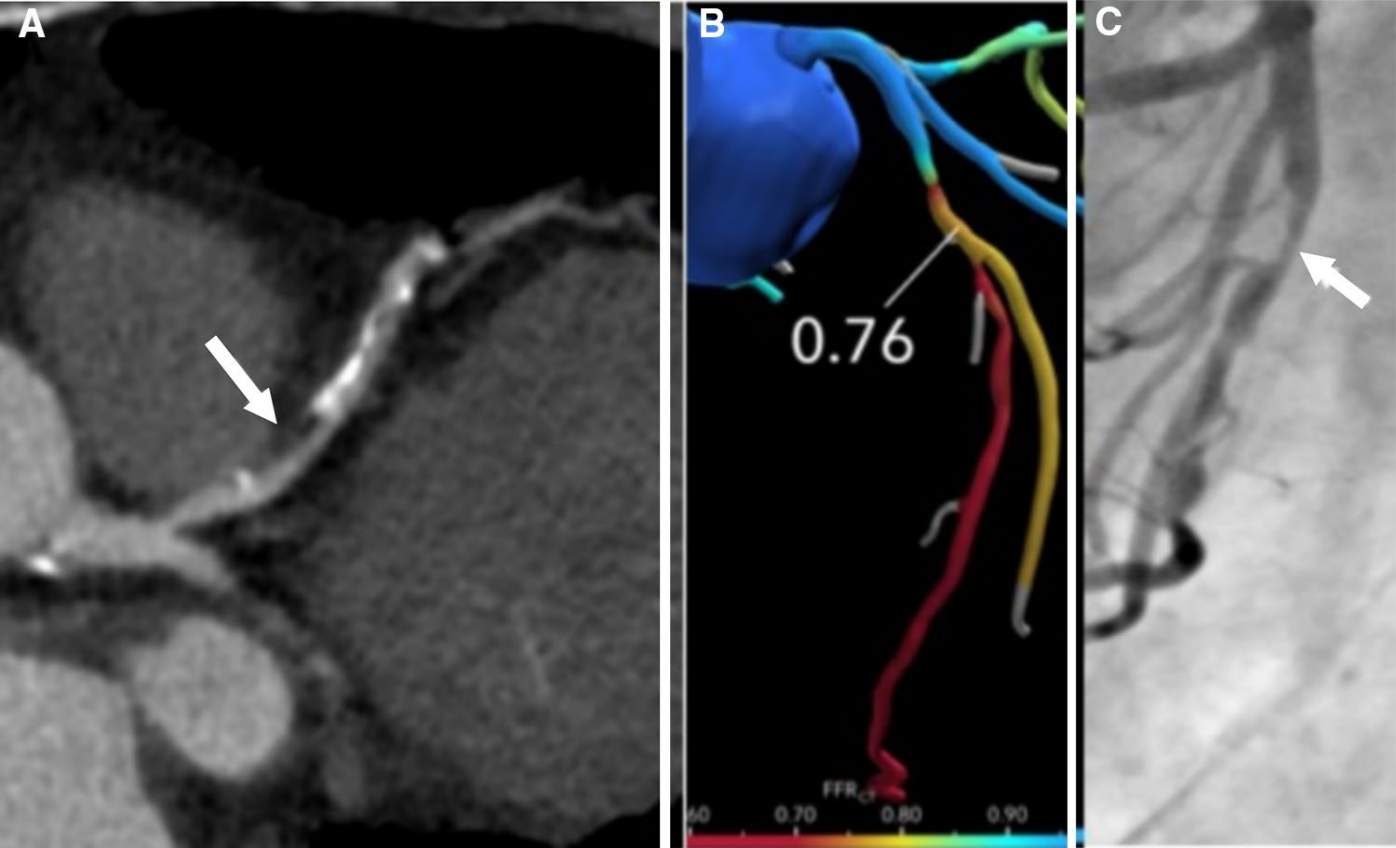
a A curved multiplanar reconstruction image of the LAD in a 66-year-old woman showing a non-calcified plaque in the proximal LAD causing moderate luminal stenosis (arrow). b CT-FFR shows a value of 0.76 in the proximal LAD, which is indicative of hemodynamically significant stenosis. c The patient was referred for ICA, which confirmed a significant stenosis with a decreased FFR of 0.65. *CT* computed tomography, *FFR* fractional flow reserve, *ICA* invasive coronary angiography, *LAD* left anterior descending artery
